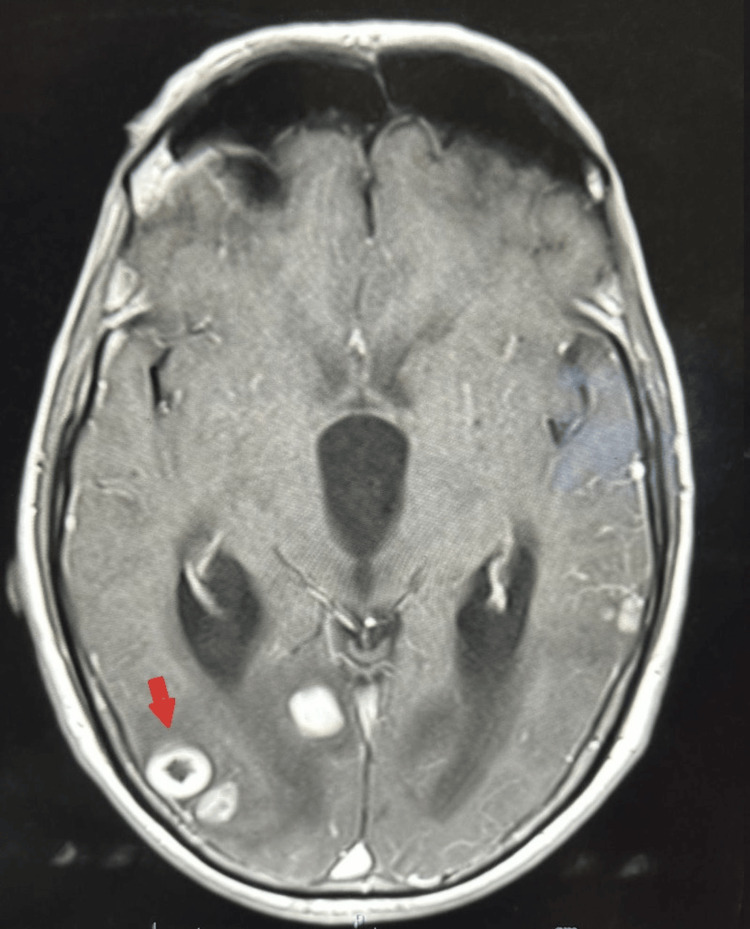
FLAIR: ring lesion with surrounding edema, consistent with tuberculoma (red arrow) FLAIR: fluid-attenuated inversion recovery
